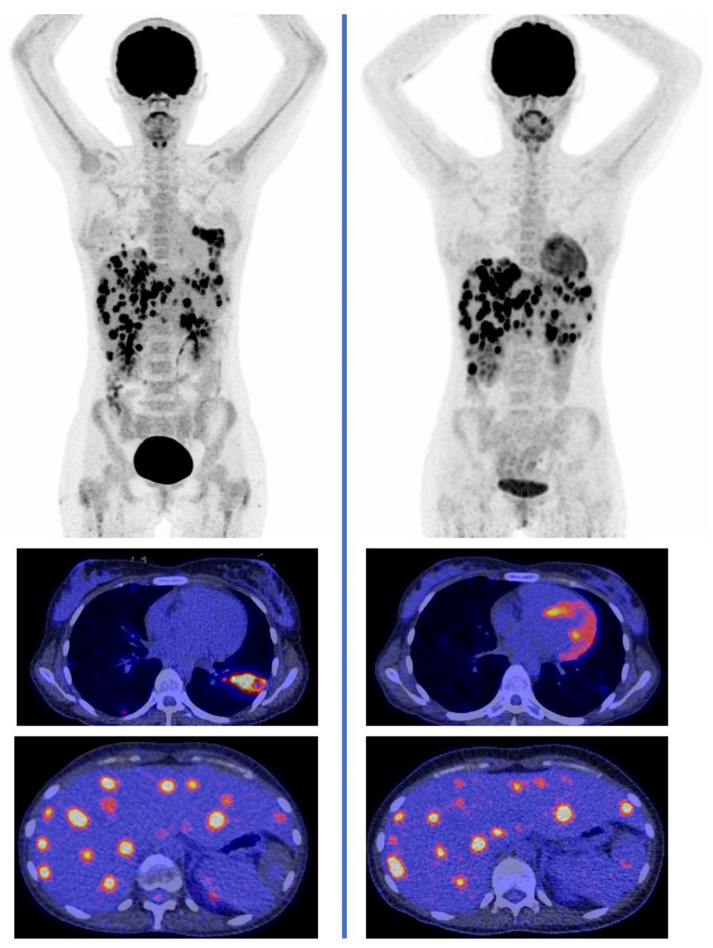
A 31-year-old female diagnosed with disseminated candidiasis after chemotherapy for acute lymphocytic leukemia. Baseline [^18^F]FDG PET/CT (left column) showed disease involvement in the lungs, liver, and spleen. Repeat [^18^F]FDG PET/CT after 3 months of voriconazole and caspofungin (right column) for treatment response assessment showed resolution of the lung lesions but persistence of the hepato-splenic lesions. Hepatosplenic candidiasis at baseline and after 3 months of therapy. The imaging finding led to a change in drug treatment.
